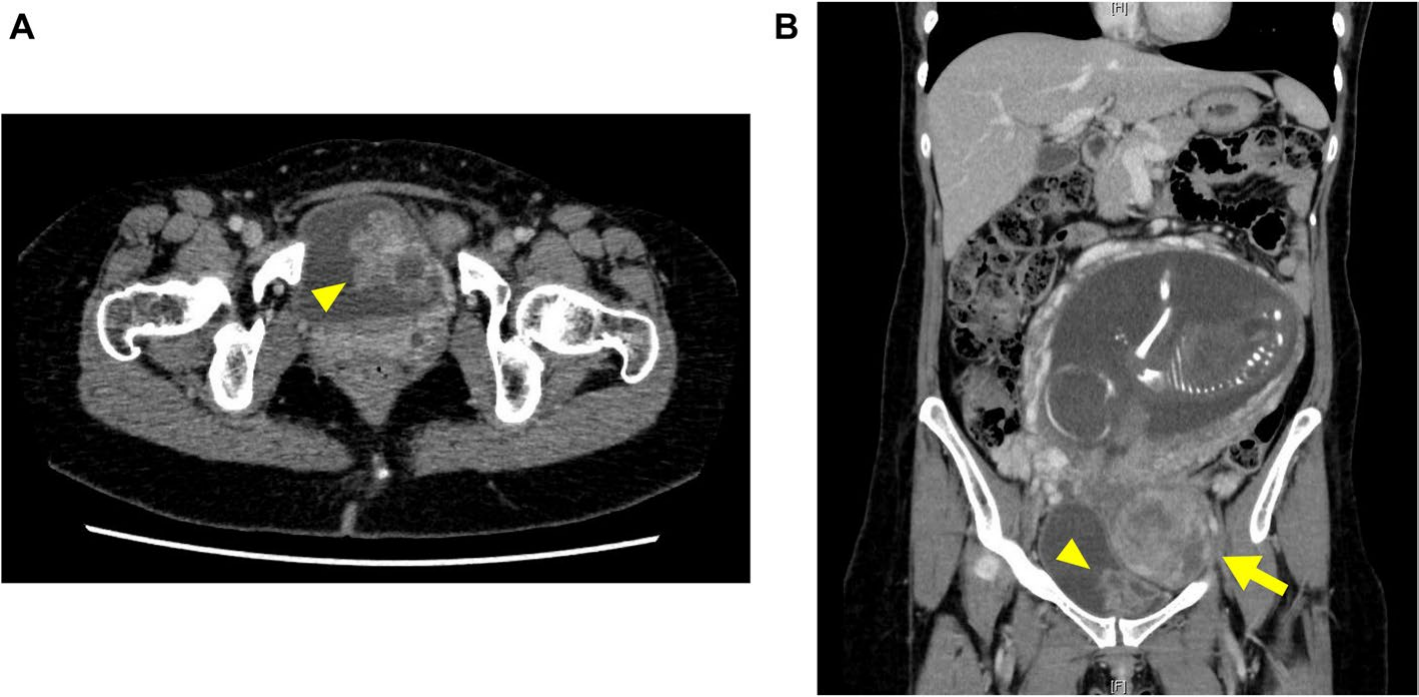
Computed tomography images at the 22^nd^ week of gestation. A Axial image of the tumor located on the left lateral wall of the urinary bladder (arrowhead). B Coronal image of the fetus, a uterine fibroid (arrow), and the bladder tumor (arrowhead)
